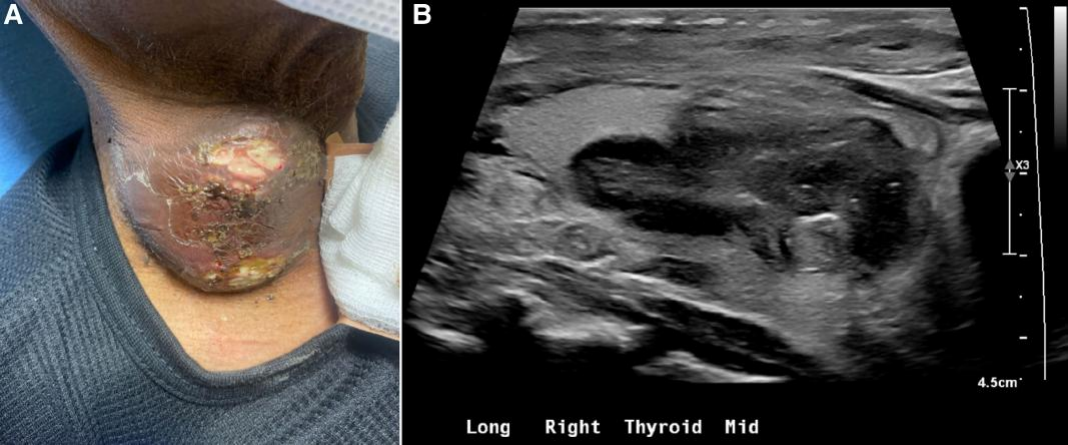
Thyroid abscess with overlying ulcerated skin and purulent drainage protruding from the anterior surface of the patient's neck (A) along with corresponding ultrasound appearance seen in sagittal view (B).
